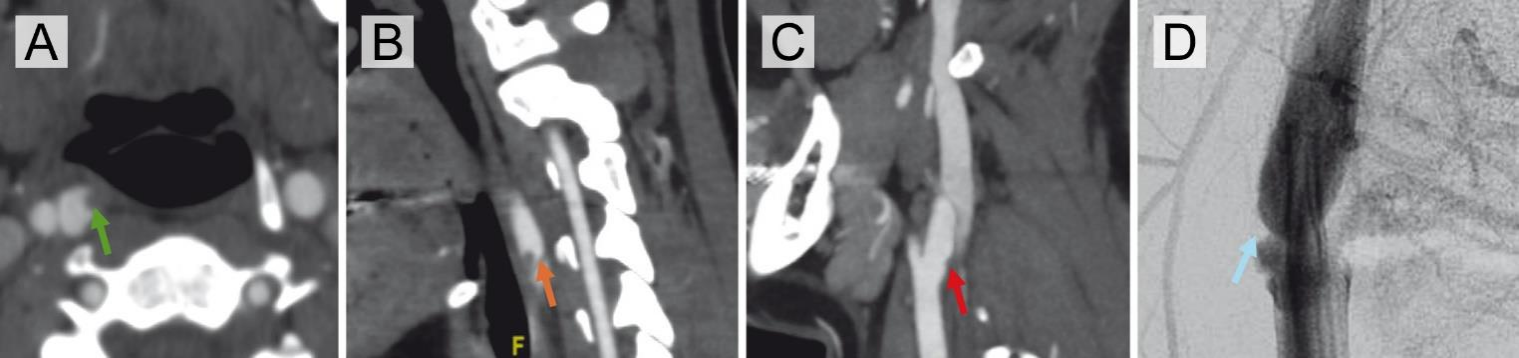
Figure 1. Imaging findings of a right carotid artery web with superimposed thrombus and interval resolution. (A) Axial CTA demonstrating a shelf-like projection along the posterior wall of the right internal carotid artery (ICA) with superimposed thrombus (green arrow). (B) Corresponding sagittal CTA confirming the presence of thrombus overlying a carotid web in the right ICA (orange arrow). (C) Follow-up sagittal CTA performed after anticoagulation shows resolution of thrombus with a small residual carotid web in the proximal right internal carotid artery (red arrow). (D) Digital subtraction angiography (DSA) confirms the presence of a subtle right carotid artery web without flow-limiting stenosis (blue arrow).
